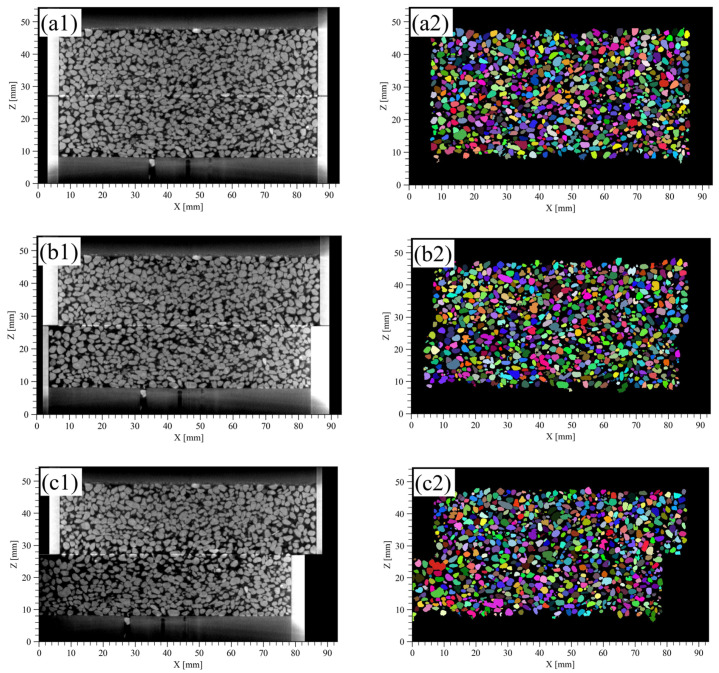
CT images and segmented images of the direct shear experiment on the vertical plane (XZ plane). (a1,a2) are the results at S0 (initial sate). (b1,b2) are the results at S2 (D_h_ = 3.0 mm). (c1,c2) are the results at S4 (D_h_ = 8.0 mm). D_h_, horizontal displacement. The left columns are CT images, and the right columns are segmented images. The boundary surface between the upper and lower boxes is at a position where the Z-axis is about 27 mm. The lower box is displaced horizontally from the +X to the −X direction.
